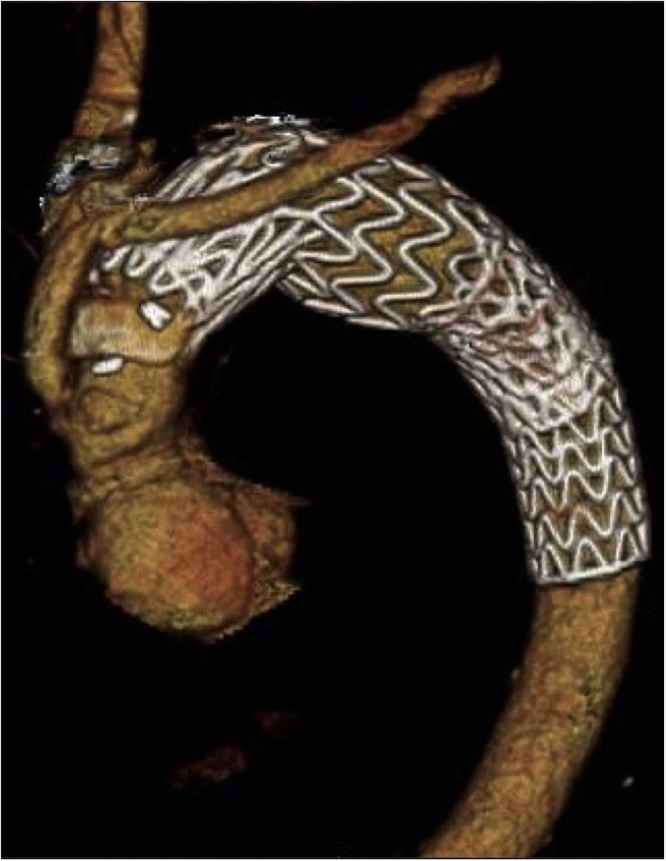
Preoperative computed tomography 3D-imaging.
